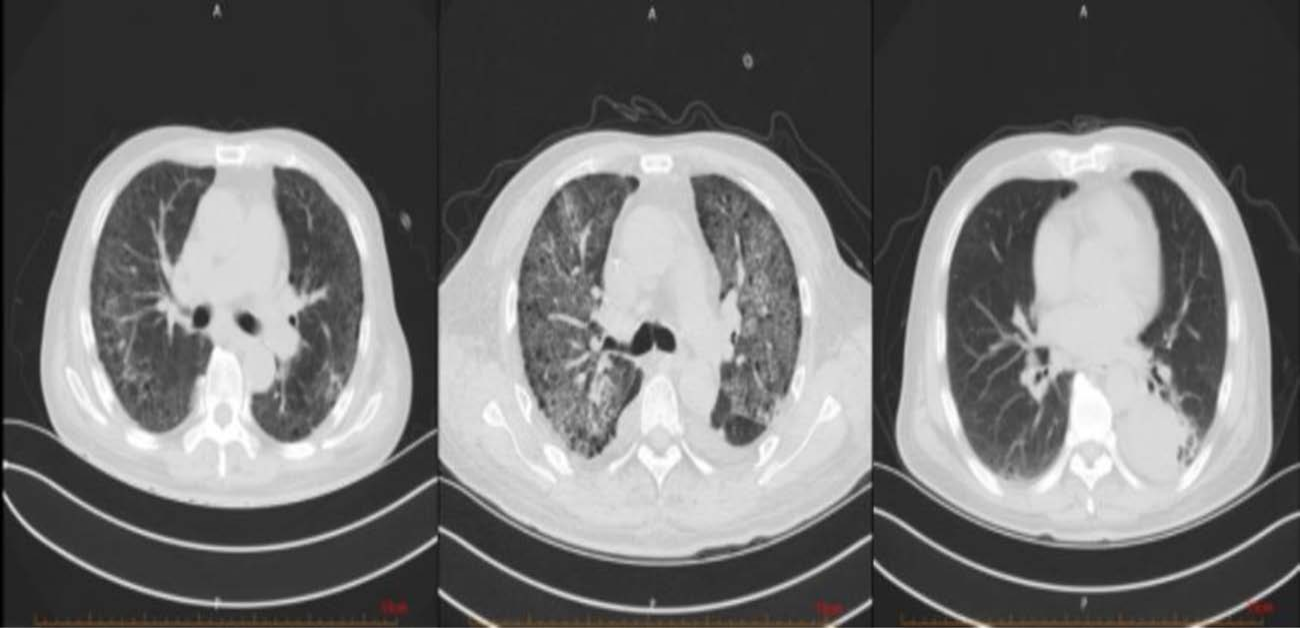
Lung CT contrast on March 4th, April 2nd, and June 12th: as the disease progressed and the diagnosis and treatment of lung lesions were carried out, the exudative lesions in the patient’s lungs decreased compared to before.
